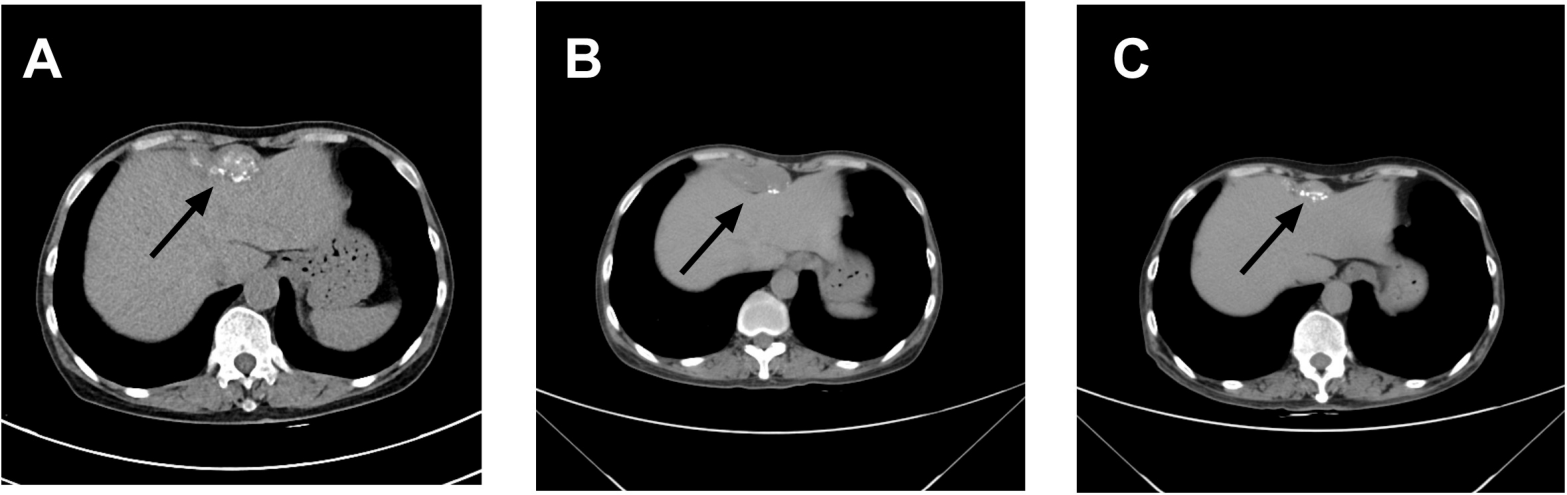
Changes in abdominal mass observed on computed tomography (CT): (A) On April 8, 2023, contrast-enhanced abdominal CT revealed an irregular soft tissue density lesion anterior to the liver, measuring 5.2 × 2.3 × 6.2 cm. A small lymph node (∼1.5 cm) was noted at the level of the umbilicus in the left abdominal cavity, with a consideration of malignant tumor. (B) On March 15, 2024, contrast-enhanced abdominal CT demonstrated a calcified mass anterior to the liver (5.1 × 2.0 × 3.6 cm) and a small lymph node (∼0.8 × 0.8 cm) at the same level within the left abdominal cavity, with a differential diagnosis of abdominal tuberculosis. (C) On October 30, 2024, contrast-enhanced abdominal CT showed a smaller anterior hepatic mass with calcification (3.9 × 1.3 cm). The lymph node at the umbilical level in the left abdominal cavity remained unchanged (∼0.8 × 0.8 cm). Arrows indicate the lesion locations.
